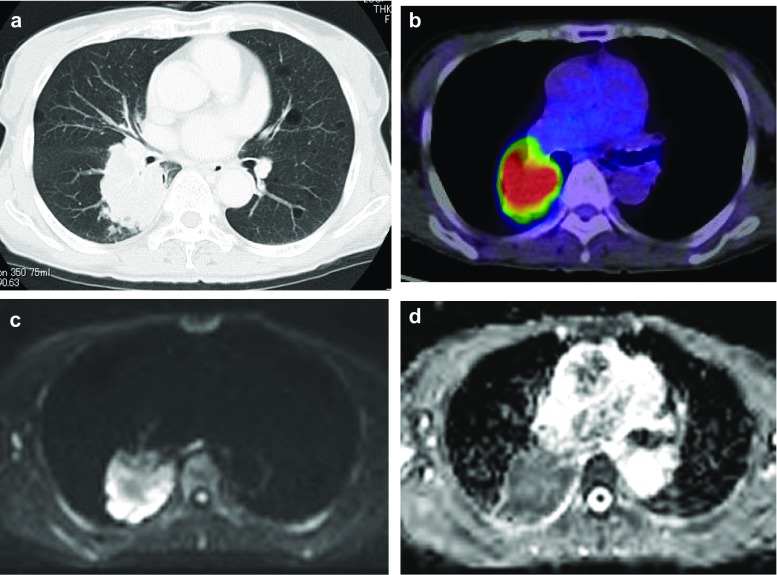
Adenocarcinoma a CT, b PET-CT, SUVmax 7.79, c DWI, and d ADCmap, ADC 1.165 × 10^−3^mm^2^/s
